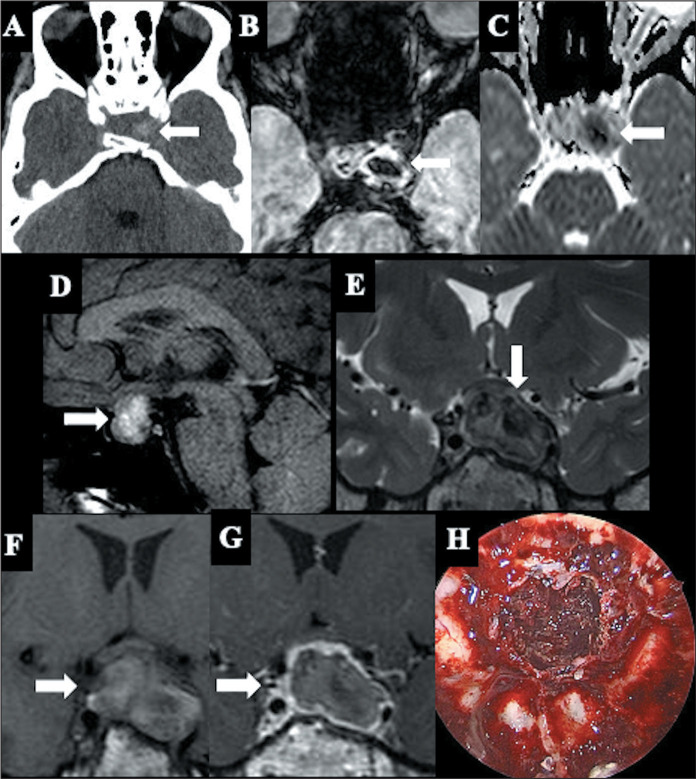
Pituitary apoplexy. A: Axial CT scan showing a hyperdense area
